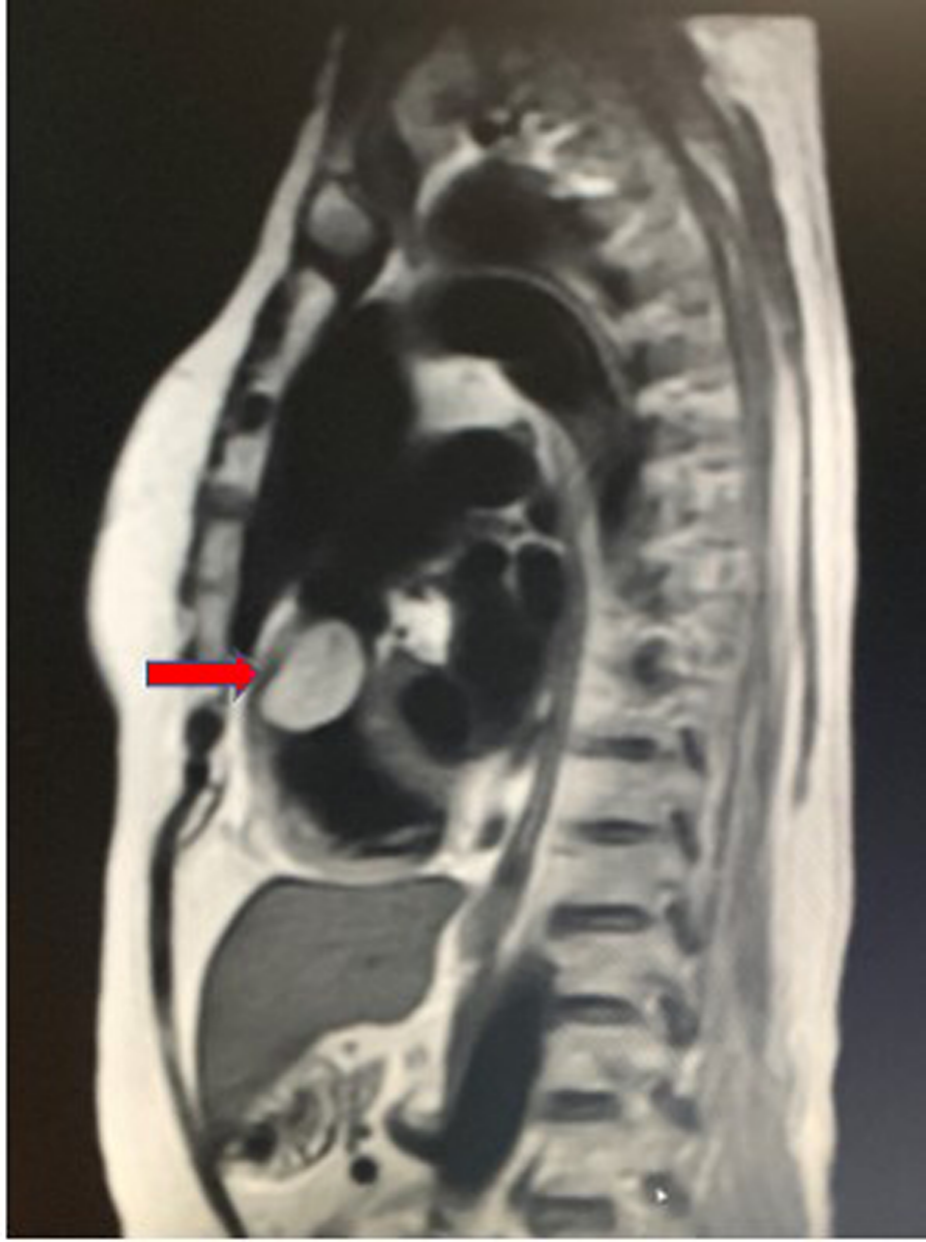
MRI demonstrated a large mass in the right ventricular cavity. The tumor was elliptic with smooth outer margin and was approximately 29 mm × 23 mm × 30 mm in size, resulting in severe stenosis of the right ventricular outflow tract.
